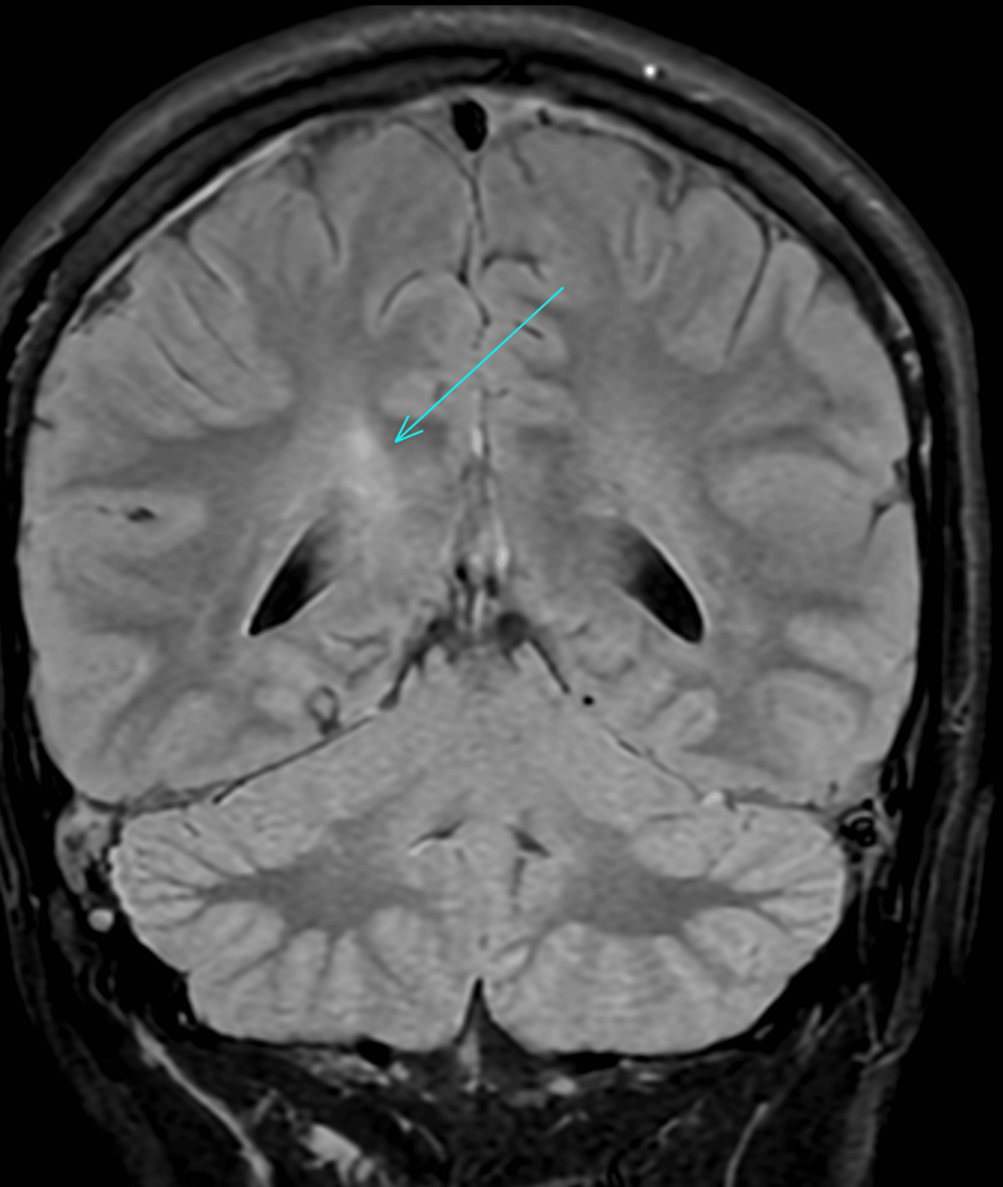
MRI head scan highlighting inflammatory changes (arrow, axial view) MRI, magnetic resonance imaging
